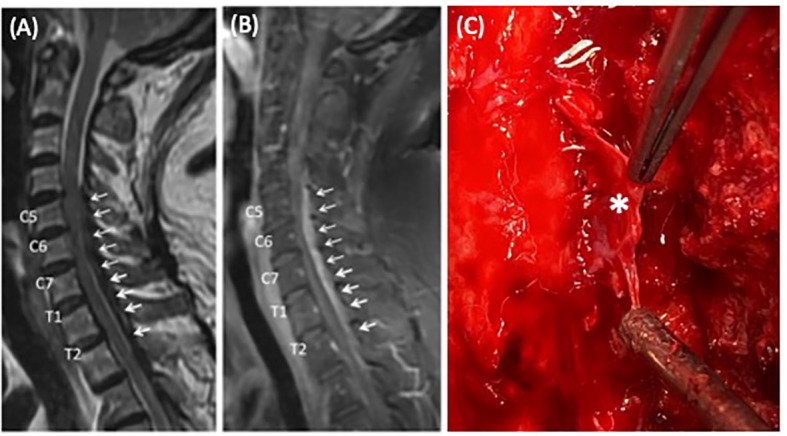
MRI of the cervicothoracic spine demonstrating severe cervicothoracic spinal cord compression and gross intraoperative appearance of the pathology. (A) Sagittal short tau inversion (STIR) image. (B) Sagittal views of gadolinium-enhanced T1-weighted imaging (T1WI) demonstrating a long-segment, homogenously enhanced tumor lying dorsally, resulting in severe compression to the spinal cord extending from the C5 to the T3 level (*arrows*). (C) The grayish sheet-like lesion (held by forceps, *asterisks*) was dissected from the spinal cord.
